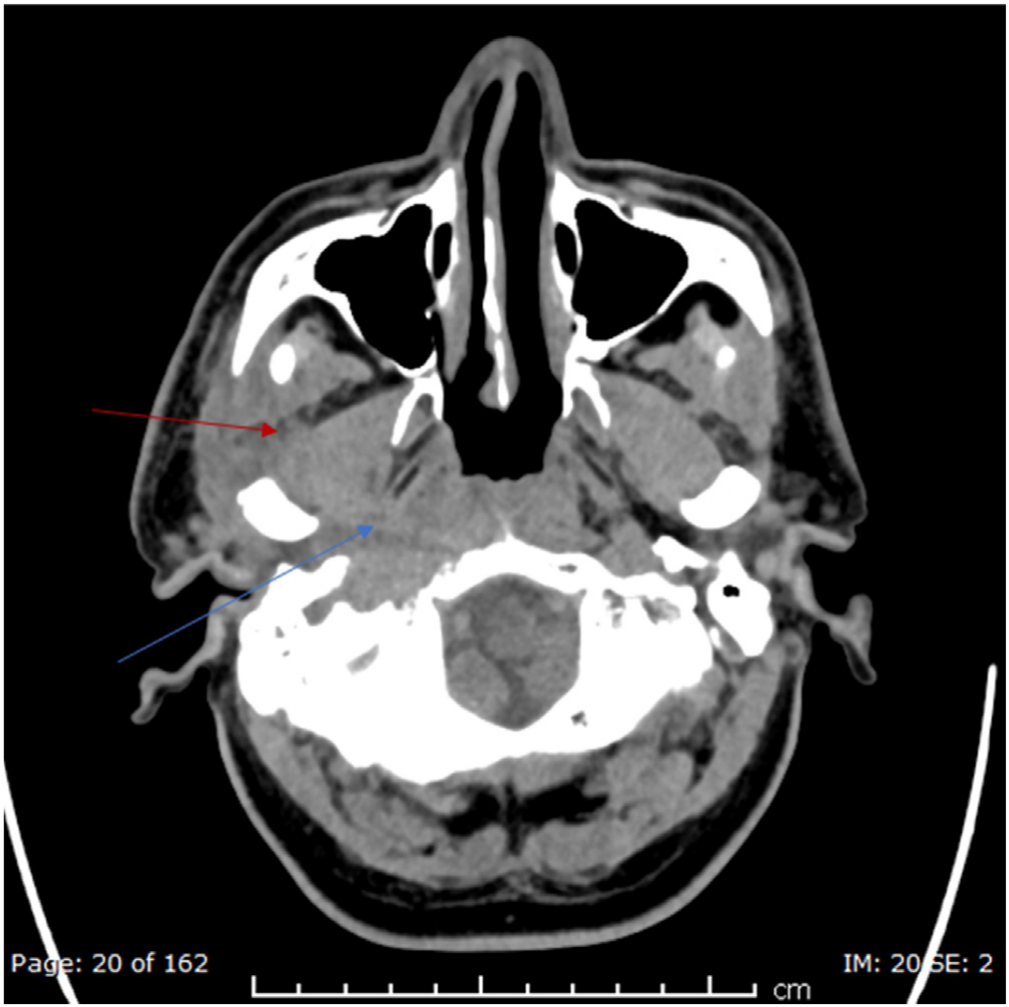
Non contrast axial CT initial scan in the emergency room demonstrated no significant intracranial pathology. However the soft tissue windows demonstrated loss of fat planes in the right parapharyngeal space (blue arrow) and masticator space (red arrow). (For interpretation of the references to colour in this Figure legend, the reader is referred to the web version of this article).
